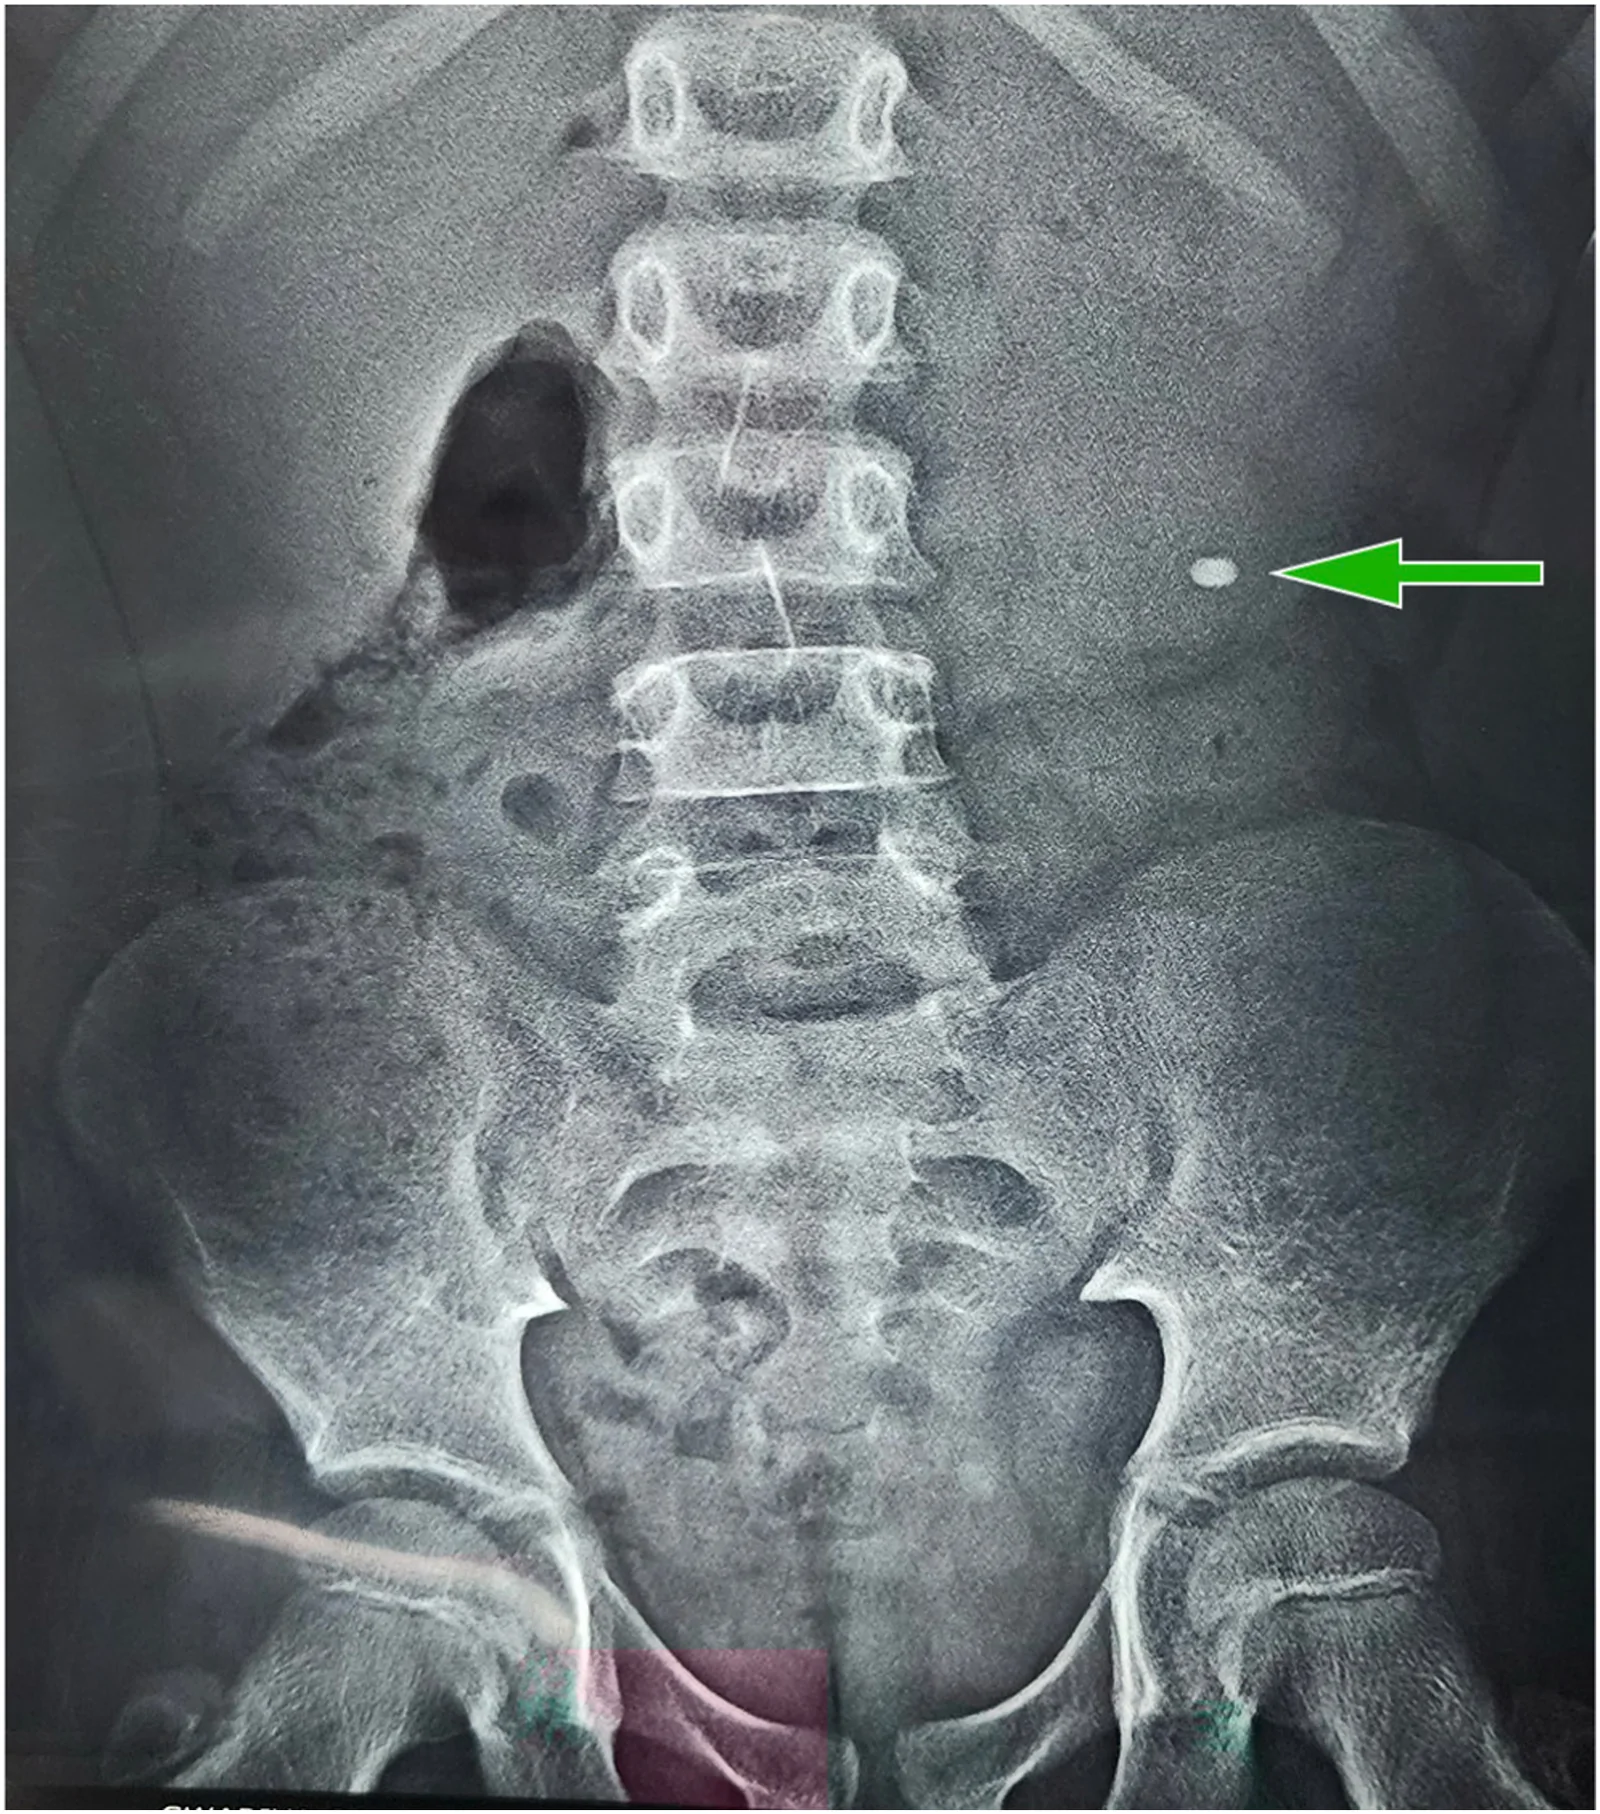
Subsequent radiograph/urogram showing the same calculus now located in the left renal region (GREEN arrow), confirming Spontaneous retrograde migration towards the kidney.
